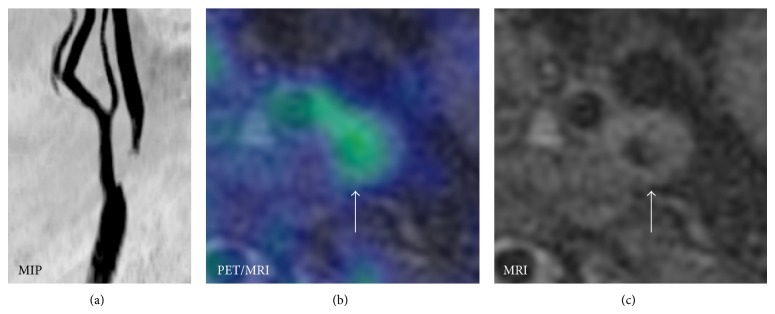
Example of simultaneous acquisition FDG-PET and MRI using biograph mMR. (a) MR angiography with severe proximal stenosis of left internal carotid artery. (b) Fusion of FDG-PET (color) and MRI with increased FDG-uptake in the internal carotid artery. (c) Transverse MR showing stenosis of internal carotid artery.
